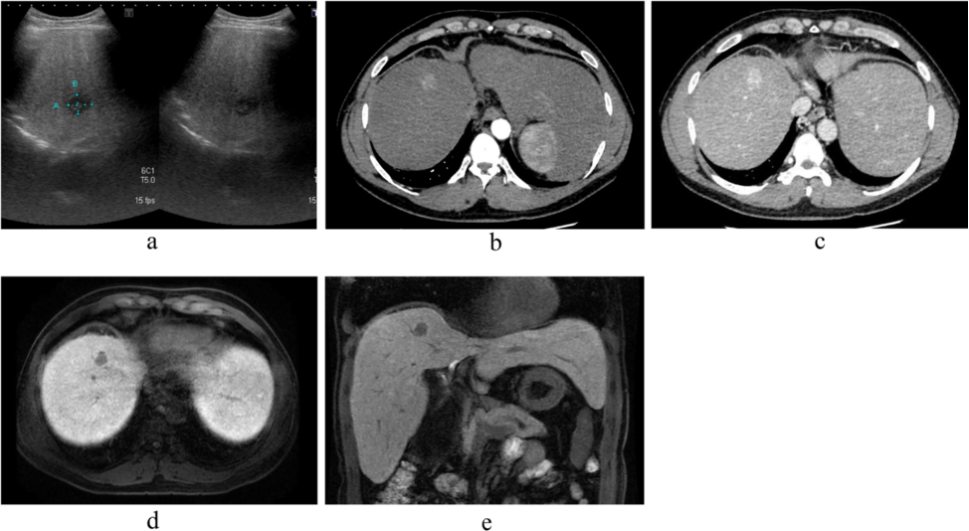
Preoperative radiological findings. a Abdominal ultrasonography showed a hypoechoic lesion in segment 8 (S8) of the liver. b The main tumor (S8) showed an early enhancement in CT, which was prolonged until the delayed phase (c). d Gadolinium ethoxybenzyl diethylenetriaminepentaacetic acid (Gd-EOB-EDTA)-enhanced MRI revealed a solitary 1.8-cm tumor, with a low-intensity signal in the hepatobiliary phase in the transverse (d) and sagittal planes (e)
